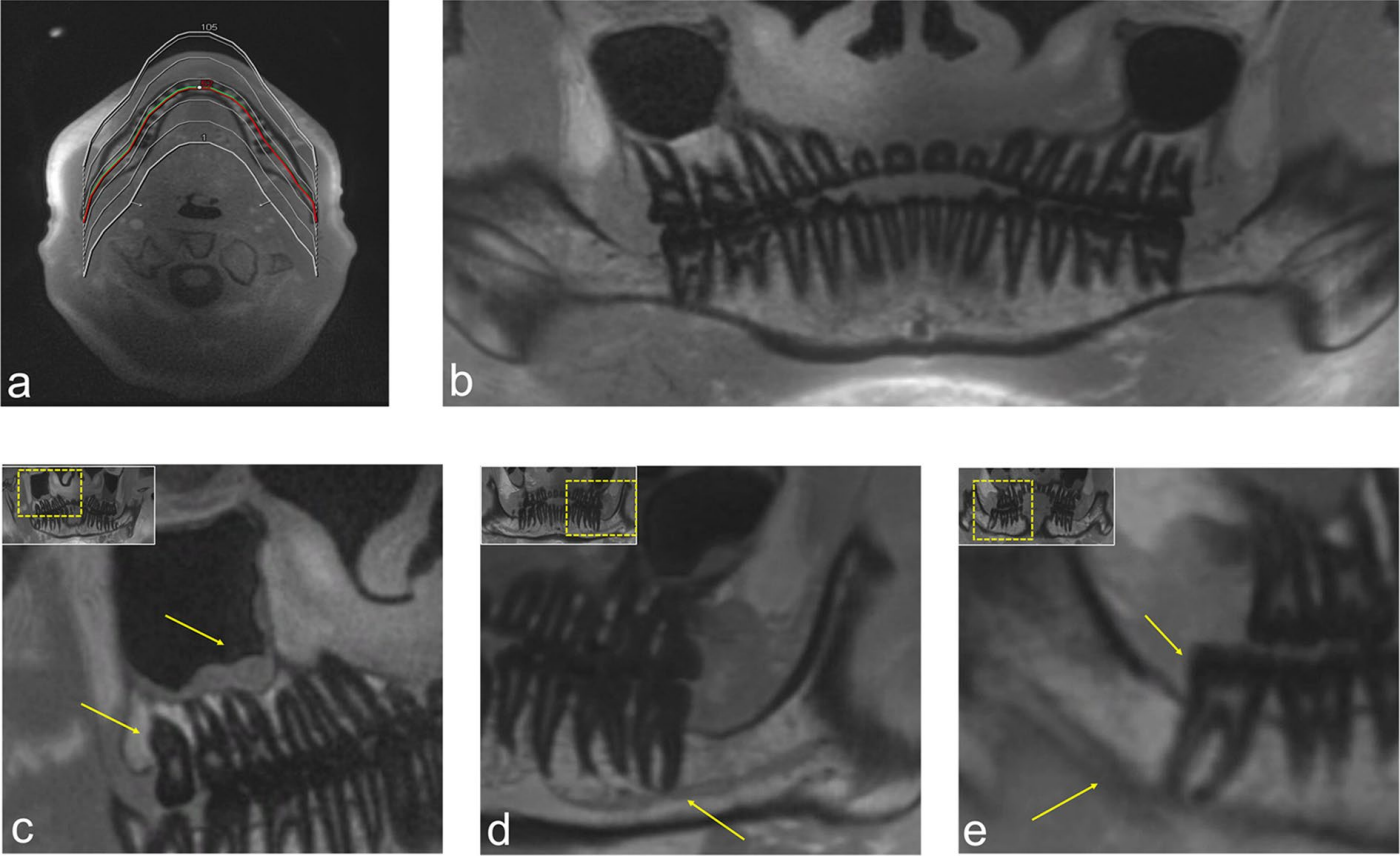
OPG (orthopantomogram) - like MRI reconstructions (MR-OPGs) from a 3D ultrashort echo time (UTE) prototype sequence for bone and teeth imaging. (a) The planning of the orthopantomogram-like MR images. (b) Overview image of a UTE MR-OPG. (c) Visualization of the positional relationship of the maxillary third molar in the first quadrant and the maxillary sinus, with a retention cyst. (d) Visualization of the course of the hypointense inferior alveolar nerve (IAN) within the inferior alveolar canal through the mandible. (e) Shows the positional relationship between the mandibular third molar and the IAN in UTE MR-OPG.
